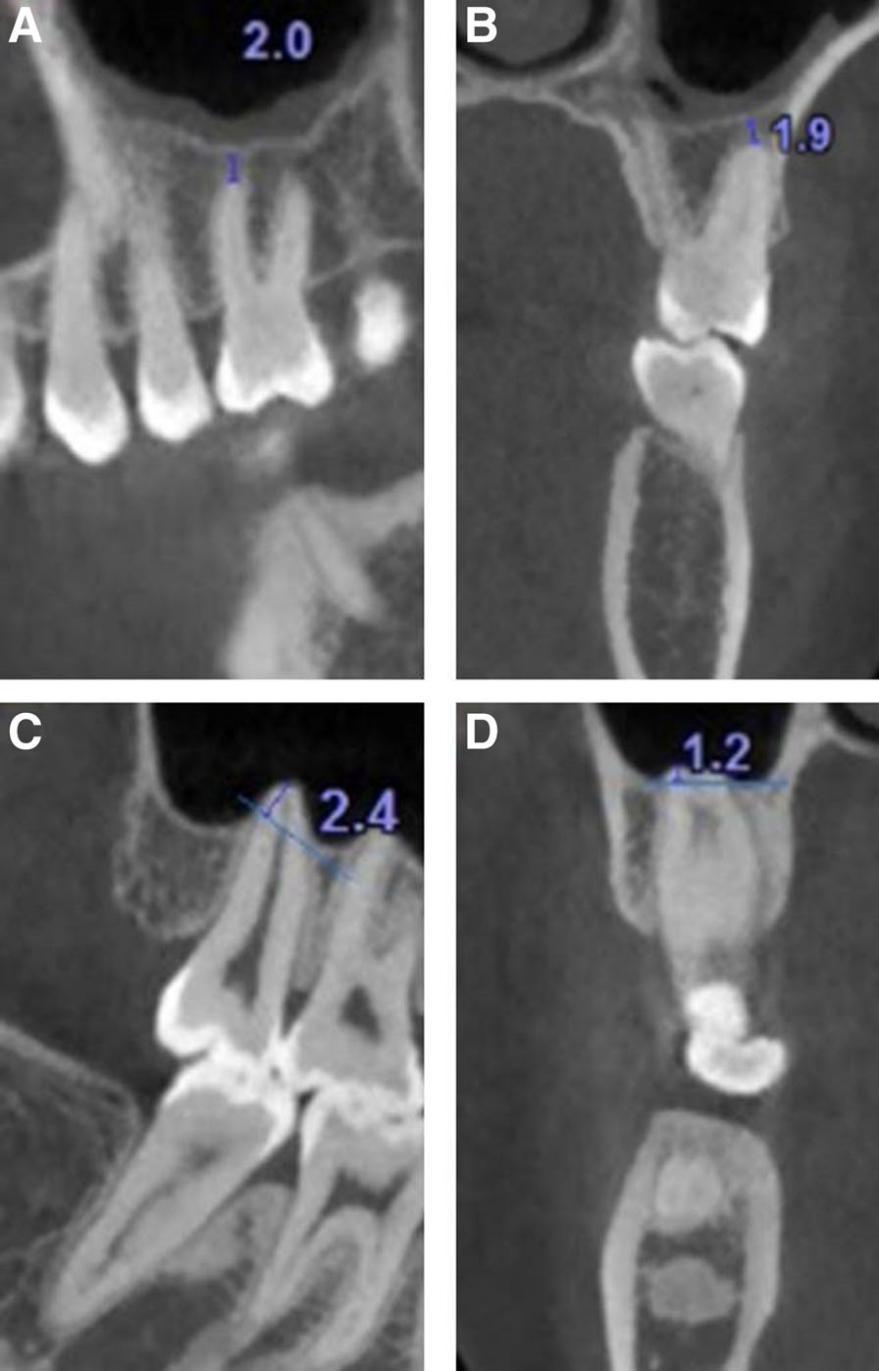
The shortest distances between the root apices and the MSFs were measured. a CBCT sagittal plane, positive value; b CBCT coronal plane, positive value; c CBCT sagittal plane, negative value; d CBCT coronal plane, negative value
